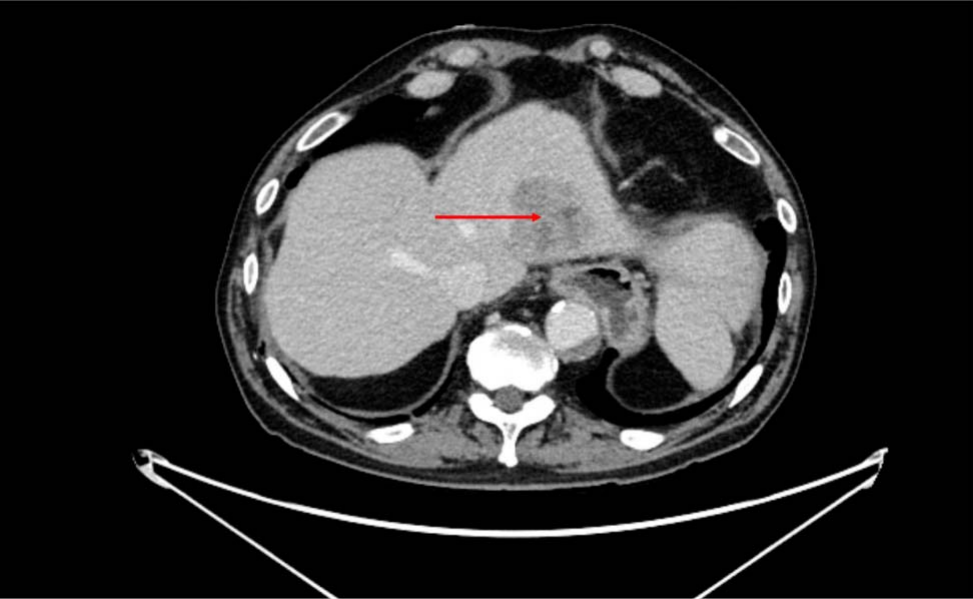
Abdominal computed tomography with contrast with a 5.0 × 4.7 cm lesion.
